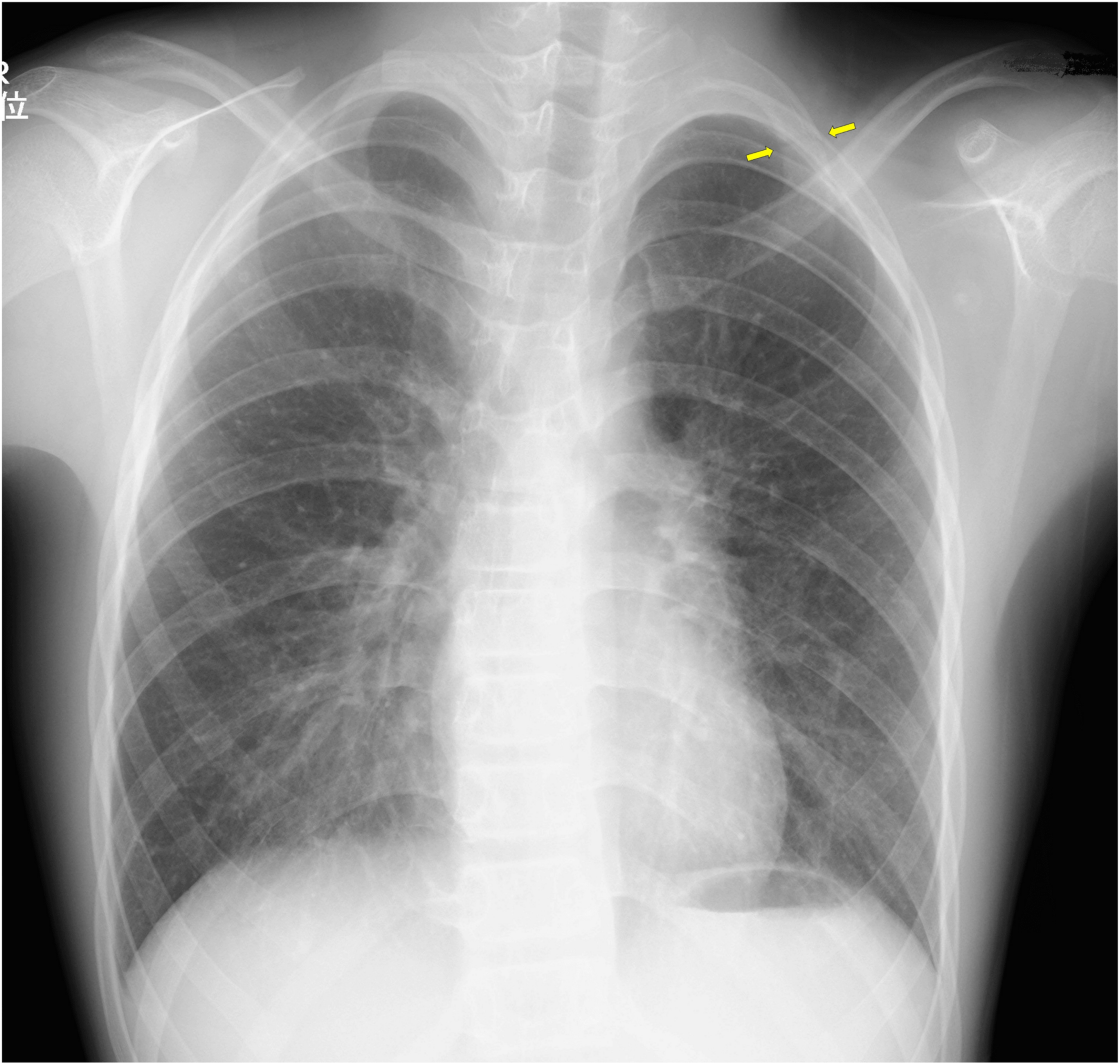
Chest radiograph. A fracture line is visible in the left first rib (yellow arrows).
